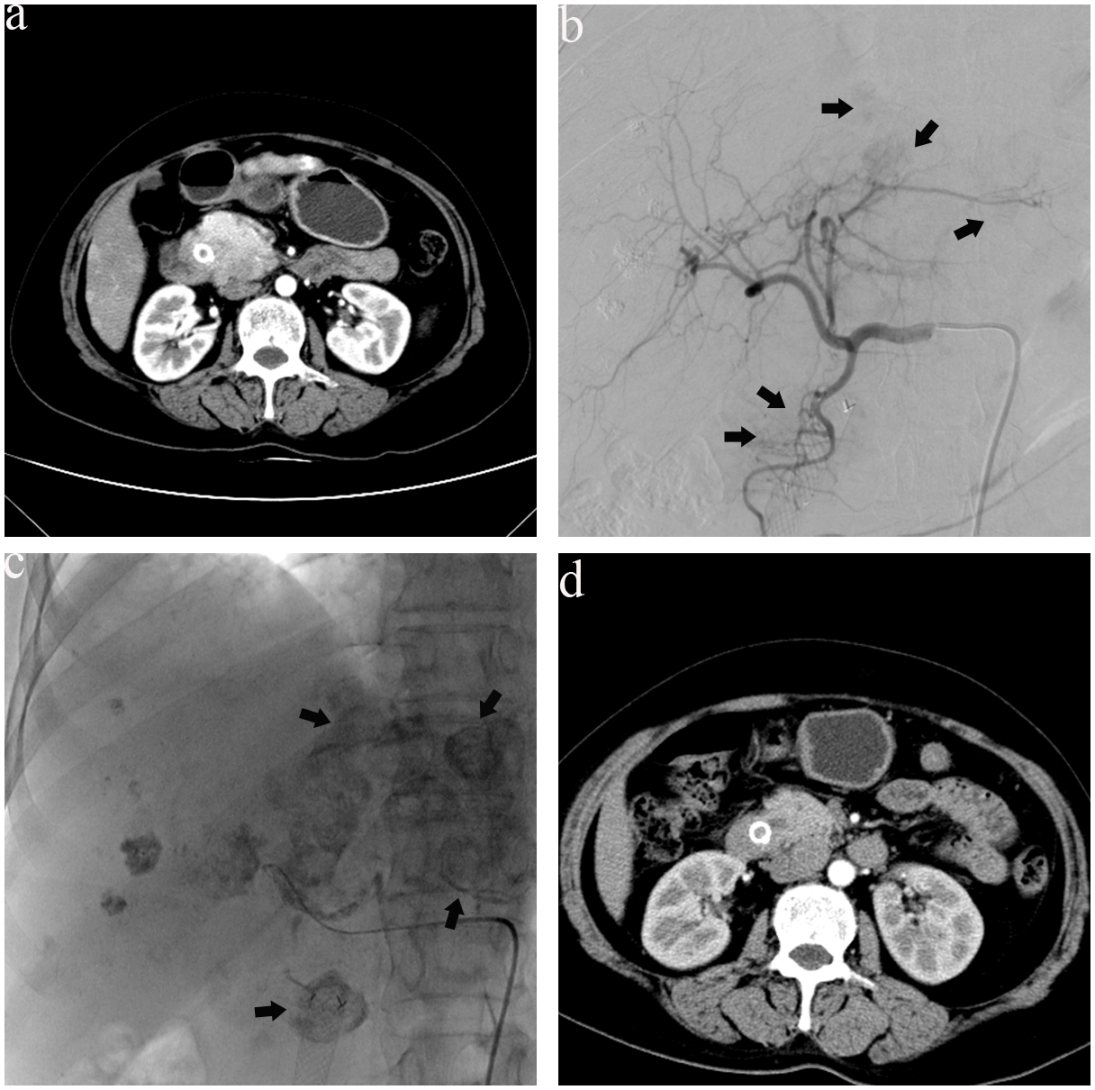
A 69-year-old man with a poorly differentiated adenocarcinoma of the middle third of the bile duct suffered stent blockage five months after stent implantation. (a) One month after intraductal RFA, CT showed the tumor was enlarged with obvious enhancement, plus liver metastases. (b) Hepatic artery angiography revealed multiple metastases nodules in the liver (arrows). (c) After the second TACE, lipiodol depositions were found in the tumor (arrows) and liver metastases. (d) CT images obtained at 3-month follow-up showed the tumor had reduced in size.
